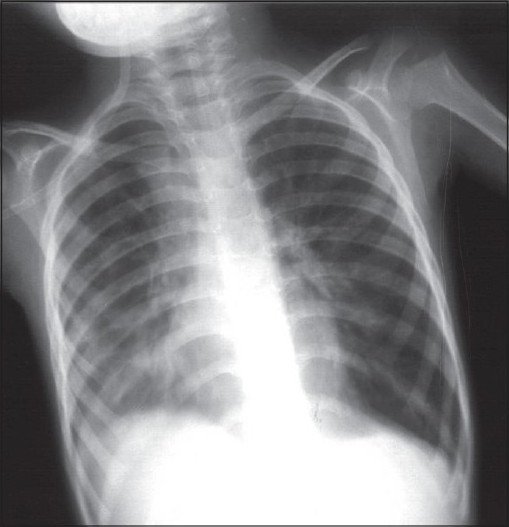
Chest radiograph at the end of two months of illness depicting hyperinflation.
